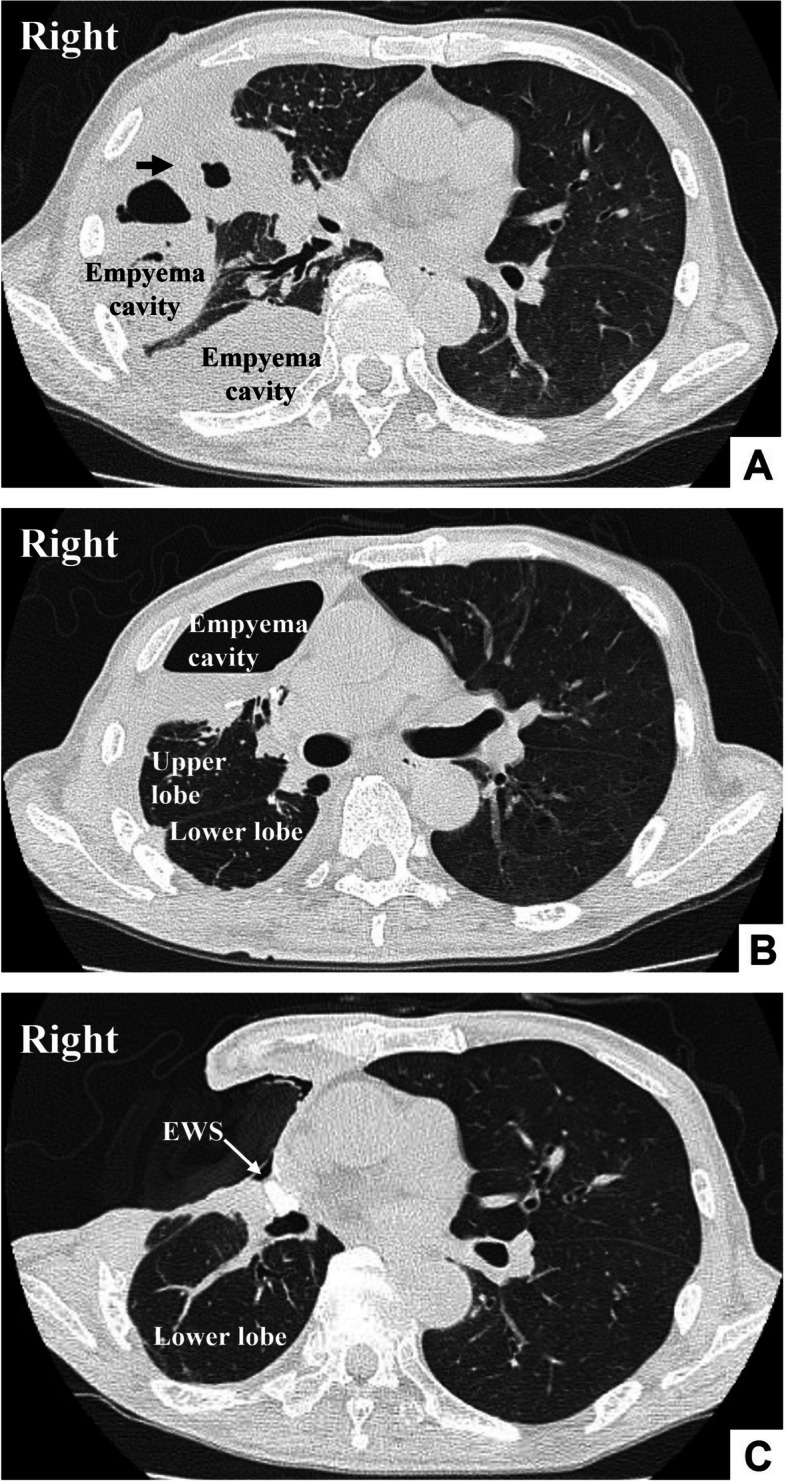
The computed tomography scan revealed a diagnosis of fistulous empyema, linked to the perforation of the right middle lobe abscess (arrow) (A). Following the thoracoscopic curettage of the right empyema cavity, we conducted a right middle lobectomy, and utilized intercostal muscle flap coverage over the bronchial stump was performed. Seven months after the surgical procedure, a bronchial fistula developed (B). After performing window open thoracotomy, our attempt at closing the bronchial fistula using multiple endobronchial Watanabe spigot (EWS) proved unsuccessful (C)
